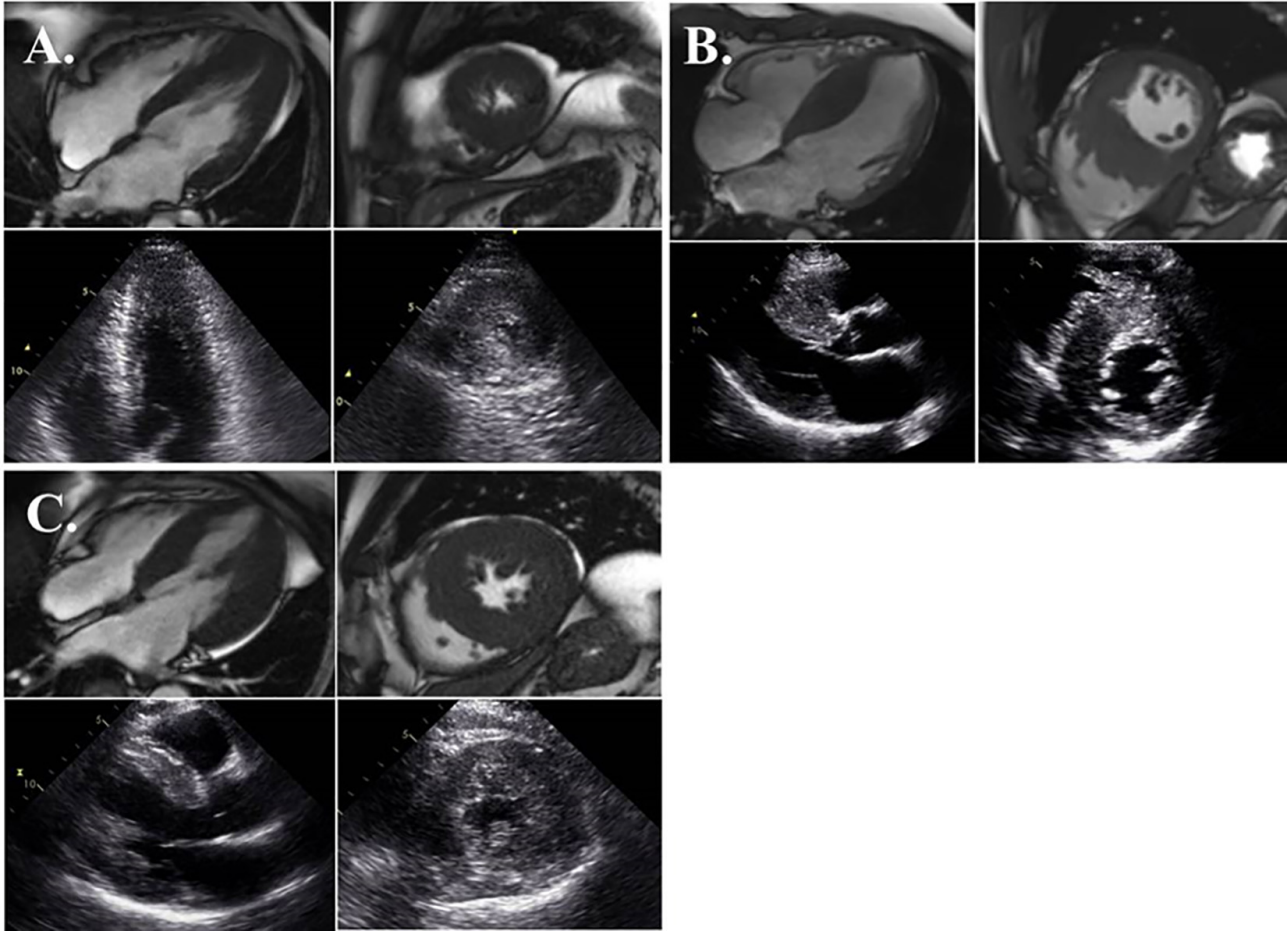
Four- and two-chamber images (upper: MRI, lower: TTE) of each HCM type: (A), apical; (B), septal; (C), diffuse.
